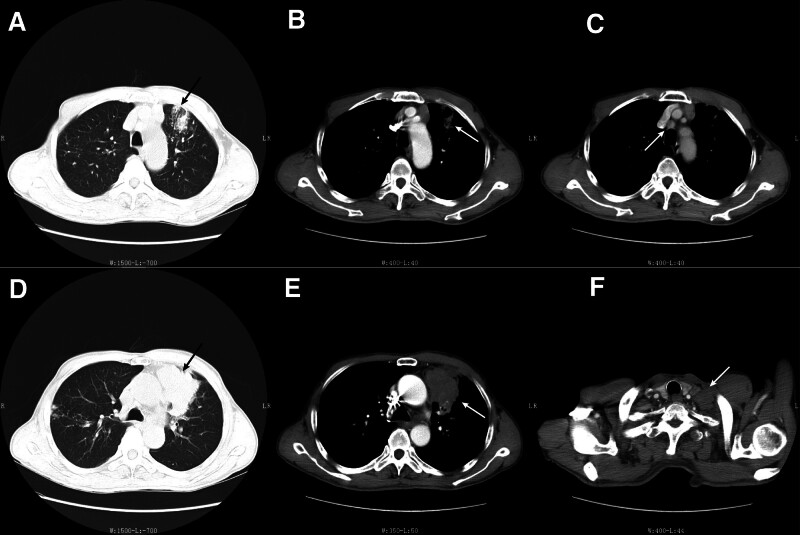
Endostar pre-use chest CT (plain + enhanced) (A) Pulmonary window at aortic arch level: patchy density shadow can be seen in the left lung; (B) Mediastinal window at aortic arch level: the left lung lesions with exudative changes; (C) Enlarged lymph nodes with marked enhancement can be seen in the mediastinum near the aortic arch; (D) Lung window at the level of tracheal bifurcation: Massive space-occupying lesion of left hilar mass; (E) Mediastinal window at the level of tracheal bifurcation: lumpy soft tissue-like density shadow with an unclear boundary and pleural adhesion. Inhomogeneous enhancement after enhanced scanning; (F) The left supraclavicular lymph node appeared enlarged and showed slight enhancement post-enhancement. CT = computed tomography.
